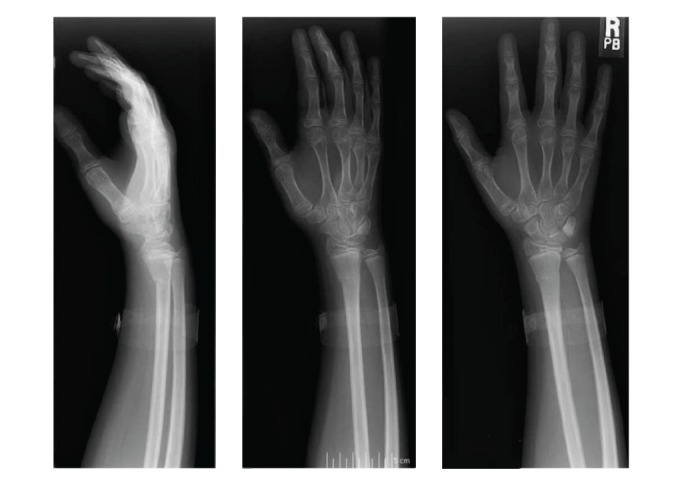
Radiographs of right wrist showing apex-dorsal distal radius buckle fracture.
